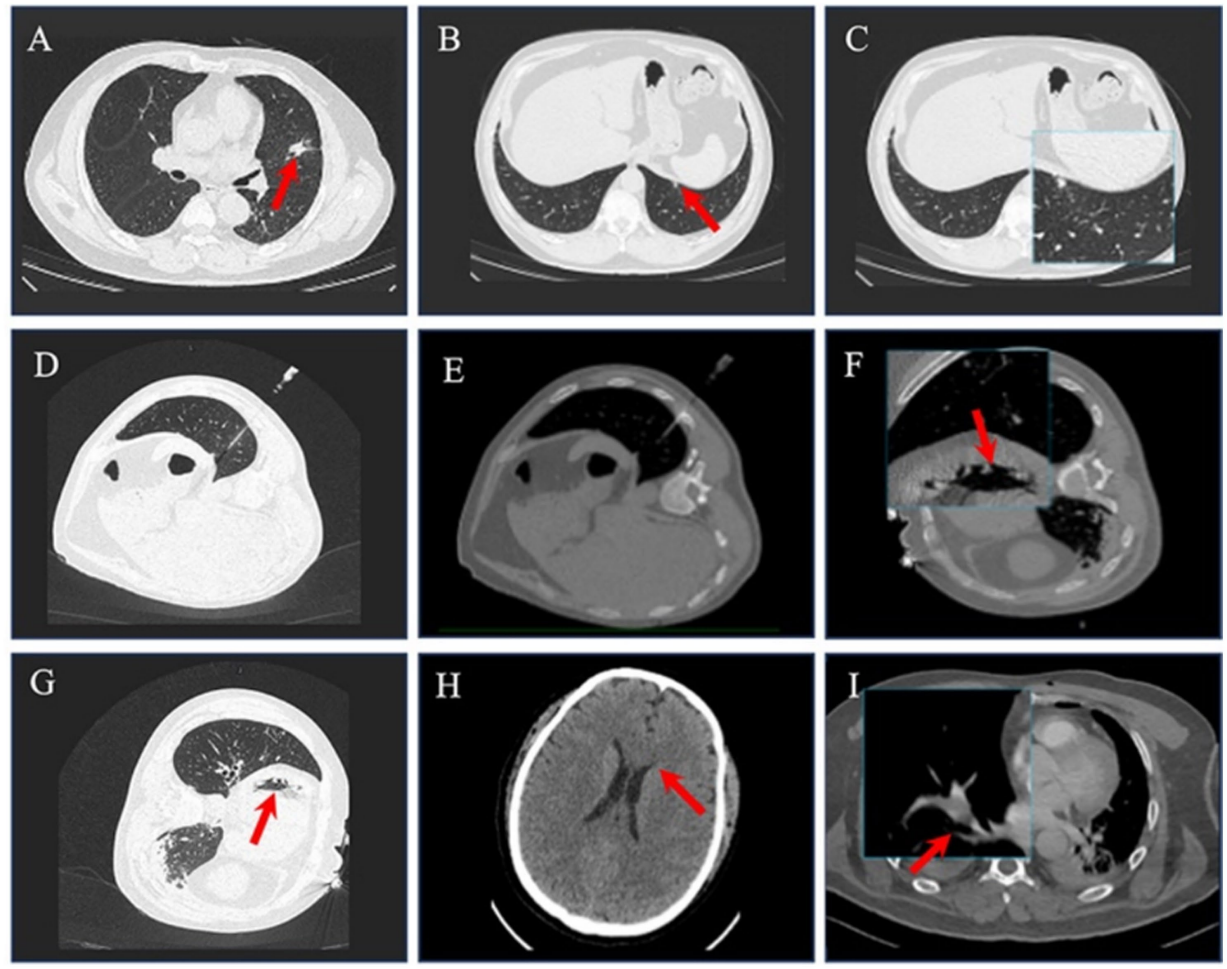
CT image of case 1. (A) CT scan a 17 × 13 mm spiculated nodule in the left upper lingular segment (red arrow), with suspected neoplastic features. (B,C) A 5 × 4 mm ground-glass opacity (red arrow and image magnification area) near the left diaphragmatic dome. (D,E) Location of the puncture needle during percutaneous pulmonary puncture. (F,G) Cardiac gas embolism following puncture (red arrow). (H) Head CT scan suspicious cerebral parenchymal edema (red arrow) after POD 6. (I) Pulmonary CTA scan embolus formation in the right middle lobe artery after POD 9.
